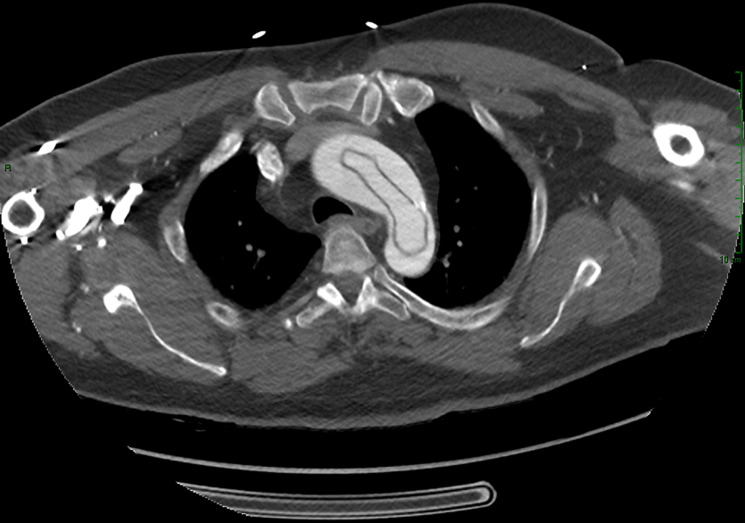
windsock sign: aortic dissection of the intimal layer on chest computed tomography angiogram
